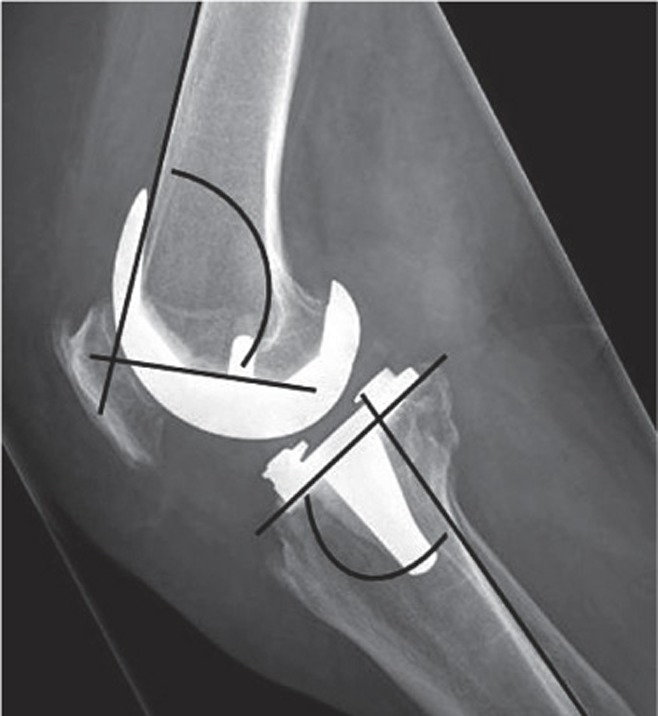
Lateral radiograph showing the LFC and LTC angles. LFC angle was defined as the anterior angle between the femoral component and the cortex of the femur. LTC angle was defined as the angle between the tibial component and the posterior cortex of the tibia.
